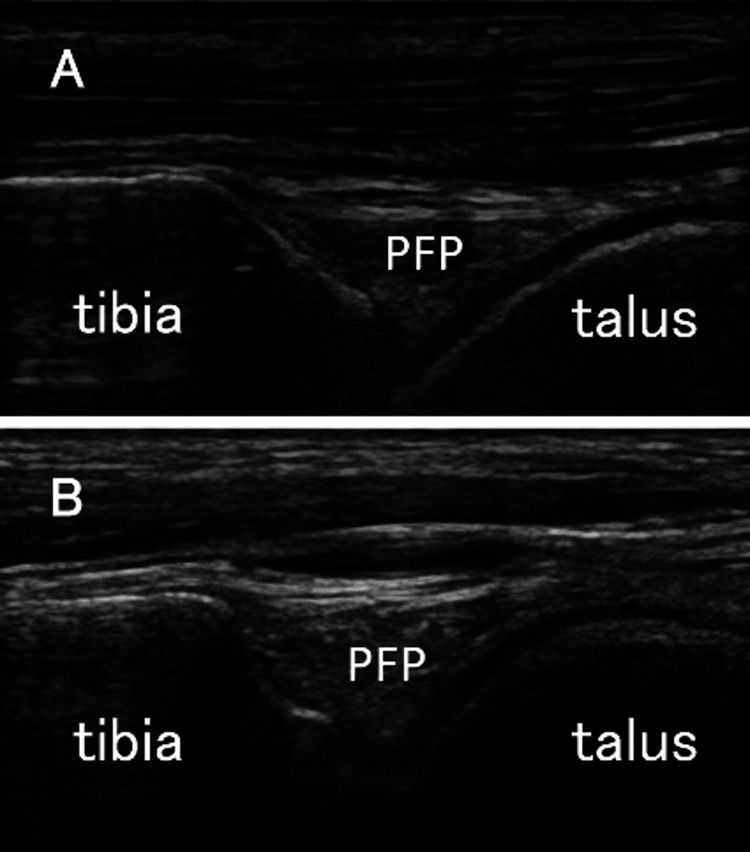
Ultrasound images of the pretalar fat pad (PFP). Ultrasound images of the pretalar fat pad (PFP) in the healthy group (A) and chronic ankle instability (CAI) group (B). The CAI group has higher ultrasound intensity and more indistinct contours than the healthy group.
